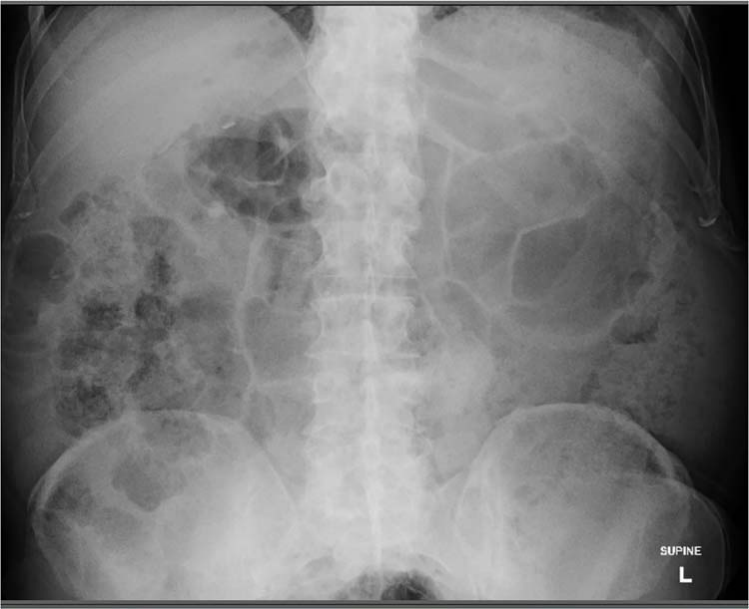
Abdominal X-ray demonstrating passage of second hearing aid.
